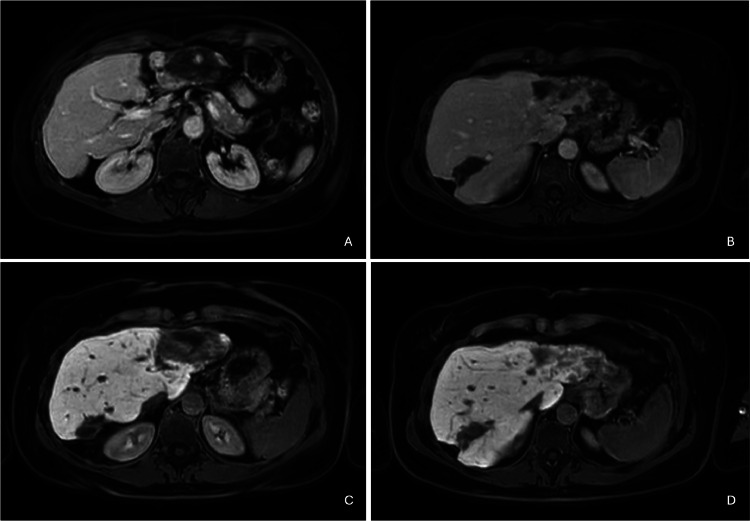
MRI performed as a three-month follow-up with hepatospecific contrast agent. Lesions at II/III and at VII hepatic segment remained unchanged on venous phase (A, B) and on hepato-biliary phase (C, D).
